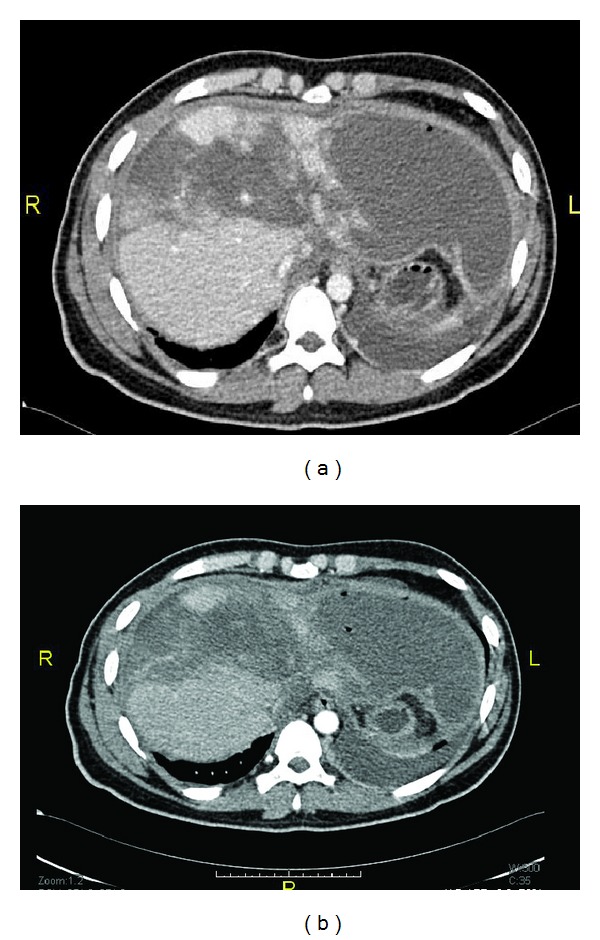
Follow-up abdomen CT (after TAE) showed massive liver necrosis with sparing of the lower part of the right lobe.
